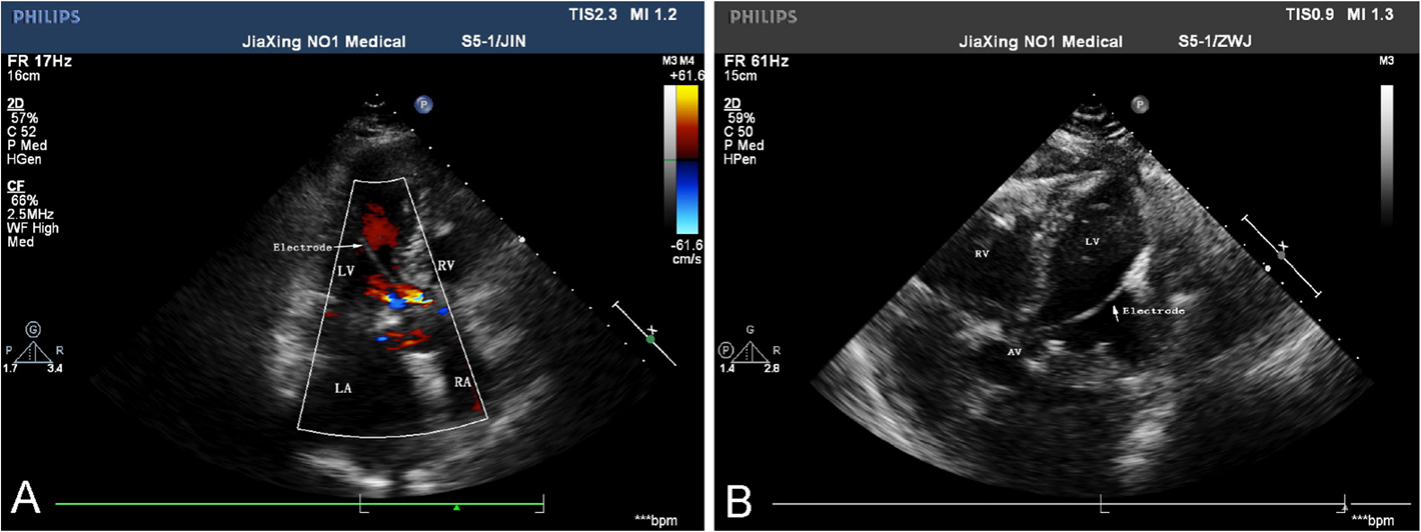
Cardiac ultrasound after operation. A Cardiac ultrasound demonstrated pacing electrode in ascending aorta and left ventricle. B Cardiac ultrasound demonstrated pacing electrode in ascending aorta and left ventricle
